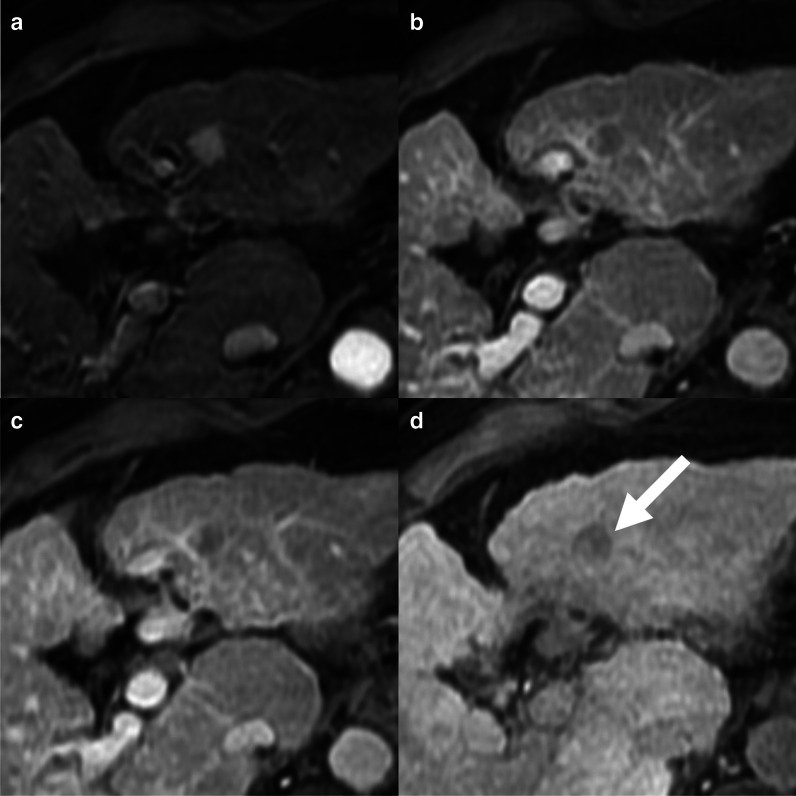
A 69-year-old man with cirrhosis and hepatocellular carcinoma. MRI acquired after the administration of gadoxetate disodium shows a 10-mm lesion with non-rim arterial phase hyperenhancement (a), non-peripheral washout and enhancing capsule on portal venous (b) and 3-min transitional (c) phases, and hypointensity on the 20-min hepatobiliary phase (d, arrow)
